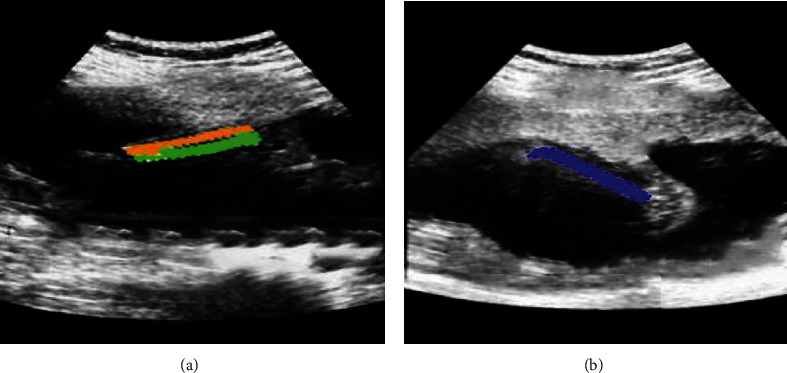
The traditional ultrasound images. (a) Fibula is marked in orange and tibia in green; (b) Femur is shown in blue.
